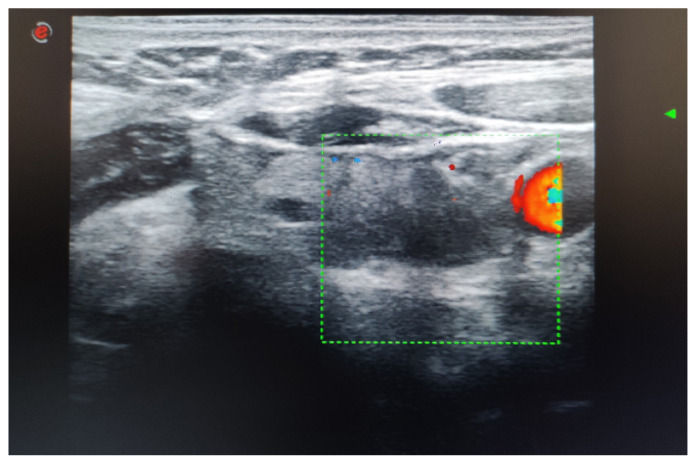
Ultrasonography of a well-defined ovoid lesion in the surgical bed after 3 months of parathyroid surgery.
